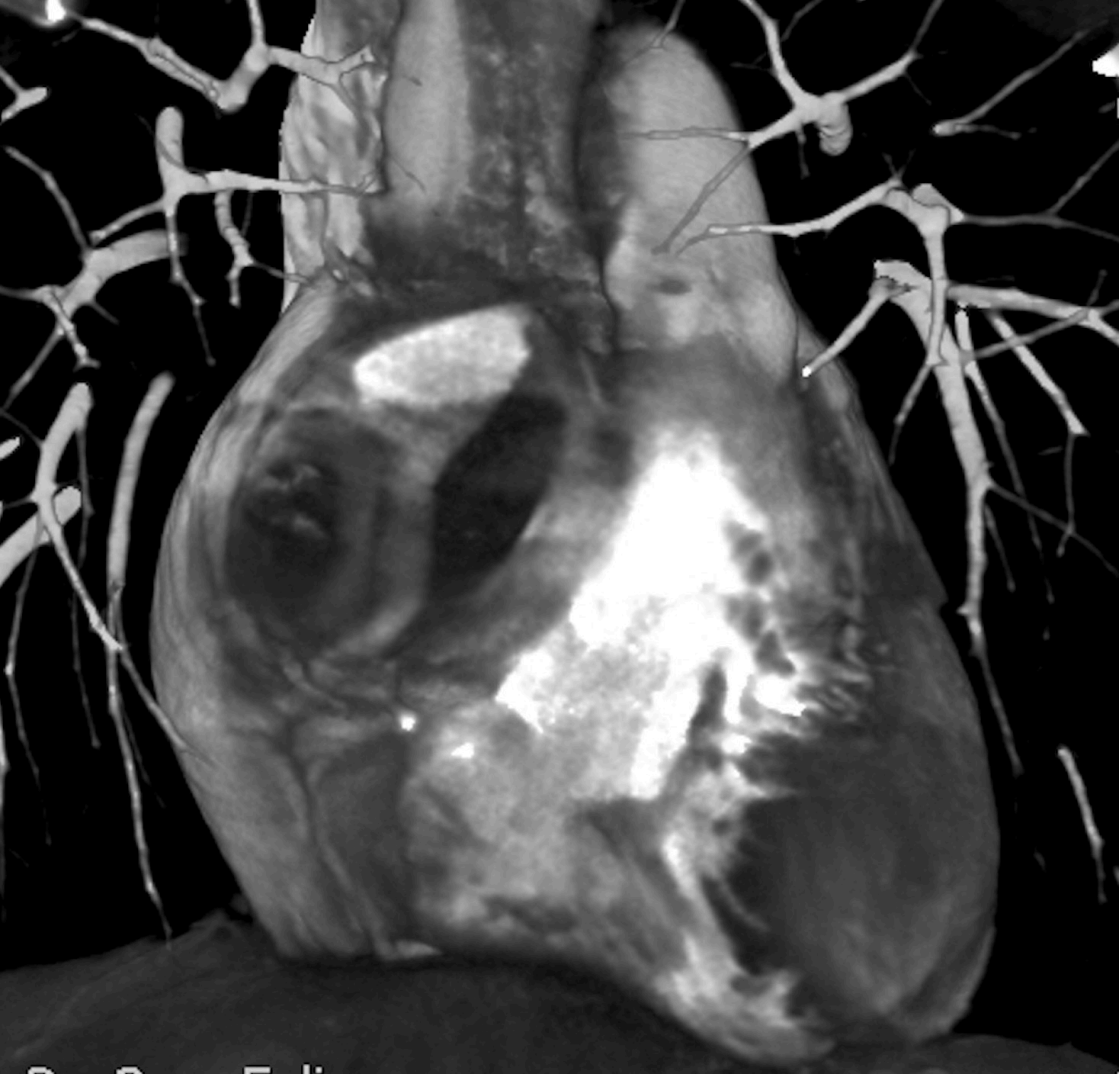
CT scan 2 MIP 3D: hypervascular mass of right coronary artery. 3D, three-dimensional; MIP, maximum intensity projection..
